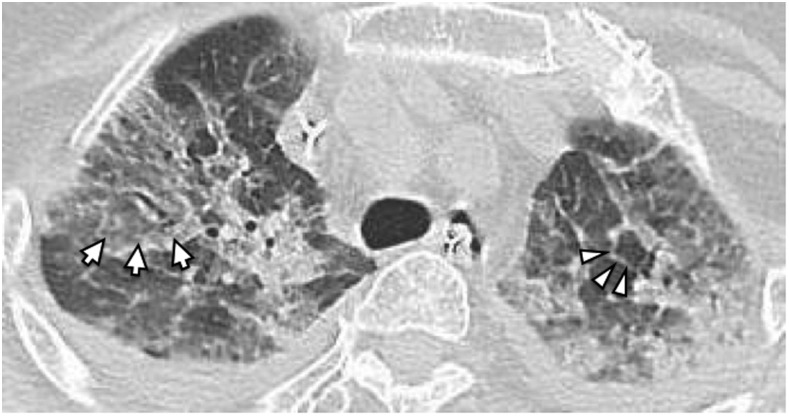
Computed tomography revealed diffuse ground-glass opacity (arrow) with interlobular septal thickening (arrowhead). It showed no findings suggestive of transmural esophageal necrosis.
